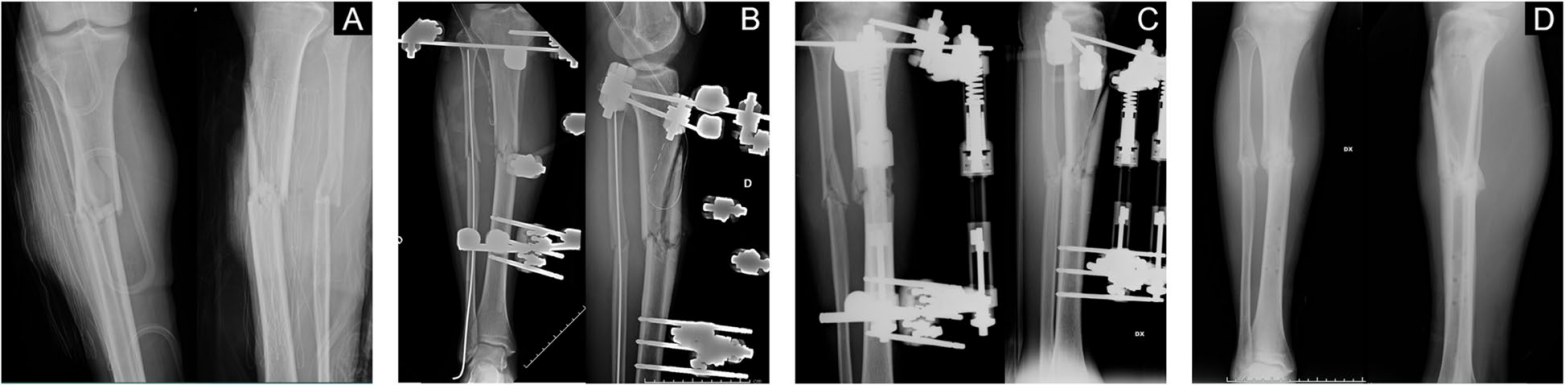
A case of a 50 years-old male who suffered from an open fracture of the right leg (A), treated with external fixation (B). Dynamization of the external fixator at 2 months from surgery and start of Teriparatide (C). Radiographic assessment after 3 months of therapy showed complete healing (D)
